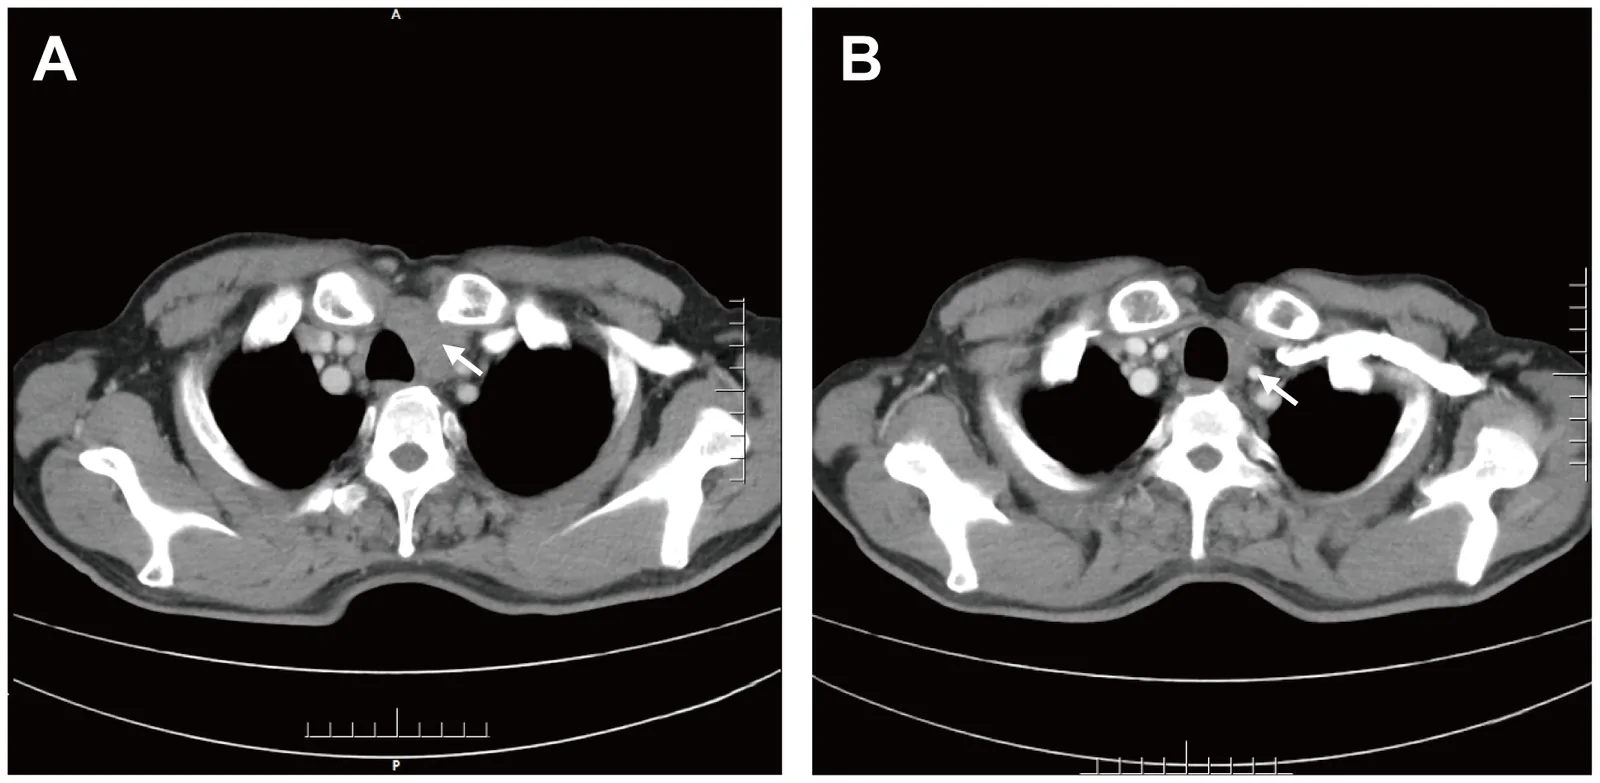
Contrast-enhanced CT of the upper mediastinum at baseline and post-treatment. (A) Baseline; (B) Two cycles of chemotherapy at the corresponding slice.
